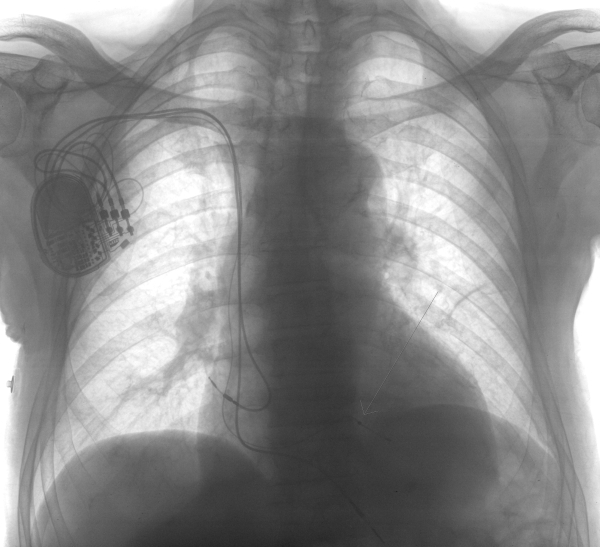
Chest X-ray showing the final re-implant from the right side
